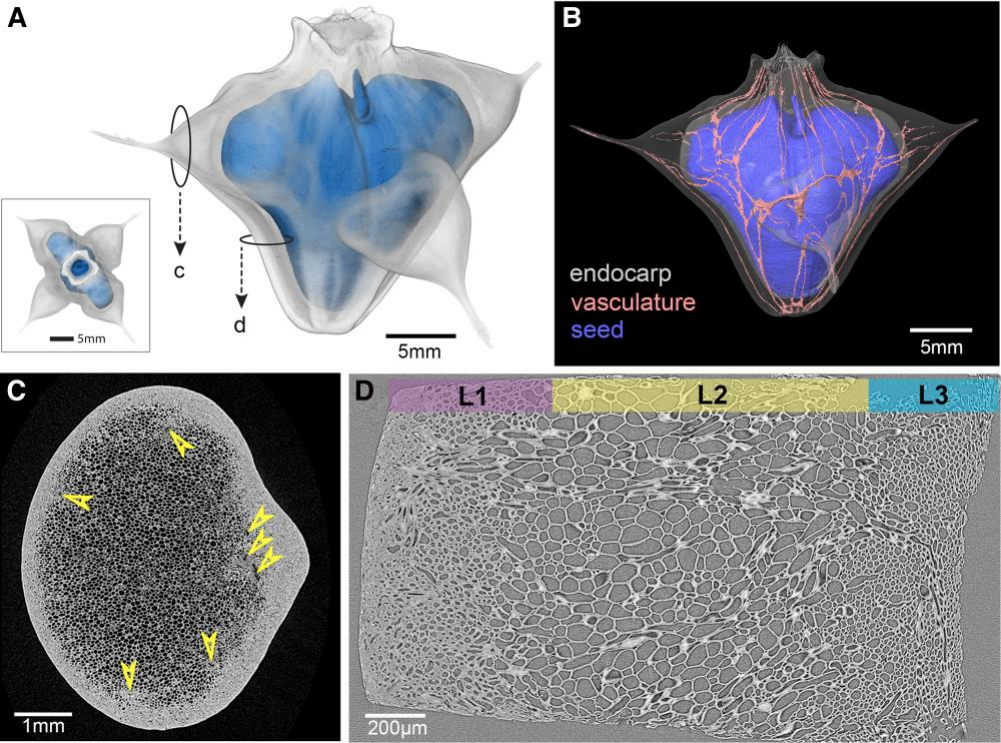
Overview of the endocarp anatomy in the mature state by means of X-ray computed microtomography. A) 3D image of the seed within the endocarp, *inset* shows top view highlighting the open structure of the terminal pore; B) 3D image of the branching vasculature within the endocarp, surrounding the seed. C) 2D image showing the density gradient in the horn tissue and arrowheads highlighting the vascular bundles; and D) the 3 distinct layers (L1, L2, and L3) showing different tissue densities (cell wall thickness and cell size) in the lateral wall of the endocarp.
